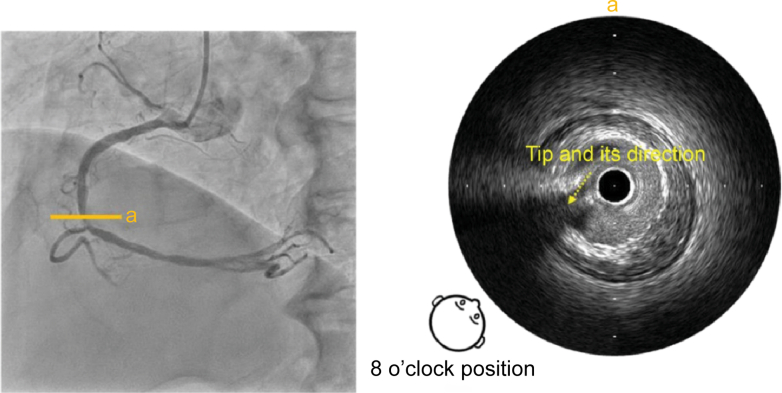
Identification of the Angiographic View on the IVUS Image IVUS image from point a. The angiographic view viewed by the operator (LAO at 50°) was observed from approximately the 8 o'clock position on the IVUS image. IVUS = intravascular ultrasound; LAO = left anterior oblique.
